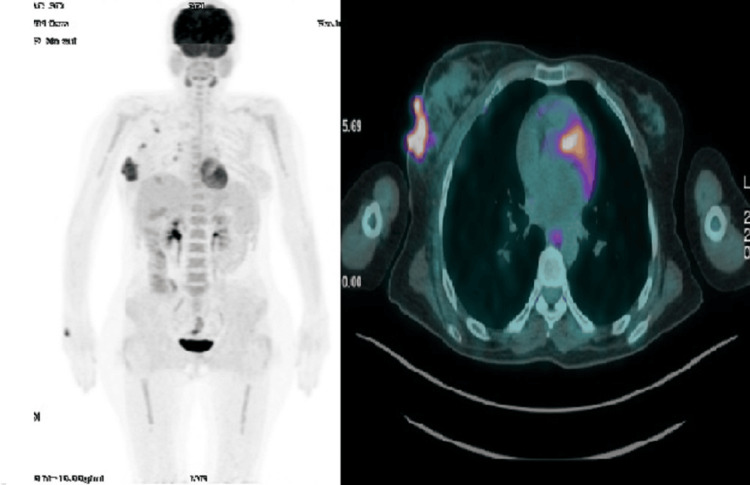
Axial and coronal reconstructions of PET scan (18F-FDG) demonstrating clear regression in size and intensity of the breast mass after the second cycle of chemotherapy FDG: fludeoxyglucose
